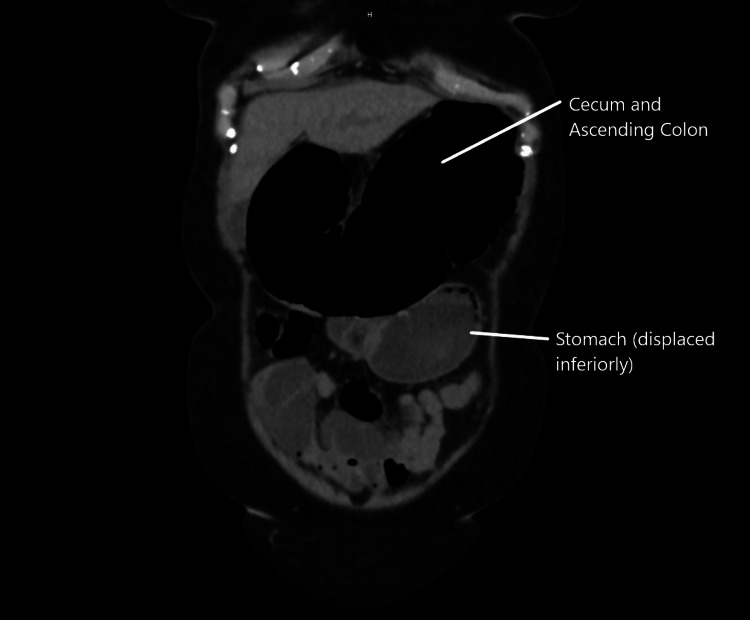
CT abdomen and pelvis without contrast. Distended cecum and ascending colon shown herniating through the epiploic foramen of Winslow (not shown). The cecum and ascending colon inferiorly displaced the stomach.
